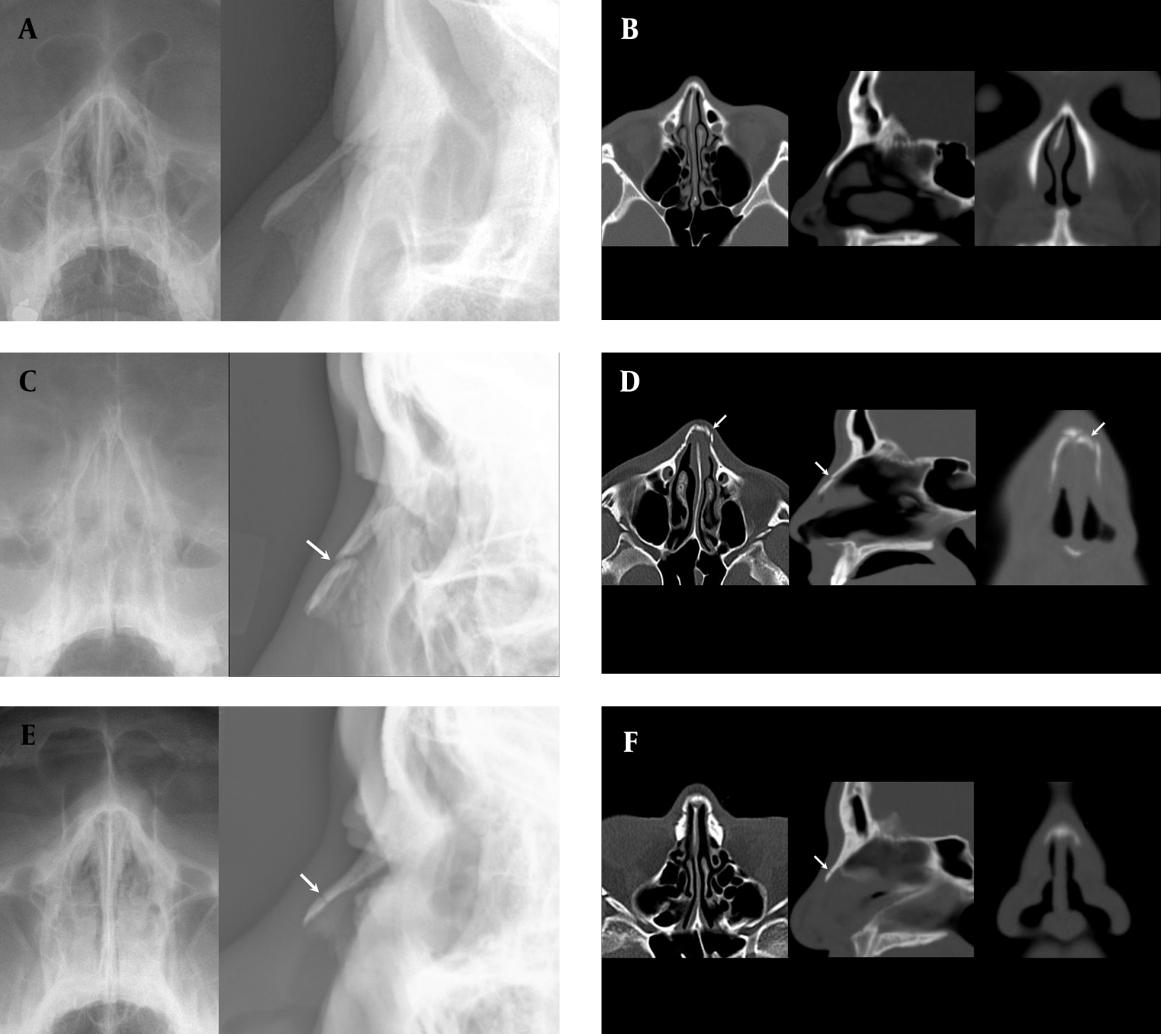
Three slices of the nasal bone are shown: A and B, a 28-year-old man with no fracture (A: coronal and lateral conventional radiography images, B: axial, sagittal, and coronal reformatted CT images). C and D, a 24-year-old man with depressed fracture (C: coronal and lateral conventional radiography images, D: axial, sagittal, and coronal reformatted CT images). E and F, a 42-year-old man with non-depressed fracture (E: coronal and lateral conventional radiography images, F: axial, sagittal, and coronal reformatted CT images) (arrows indicate nasal bone fracture).
